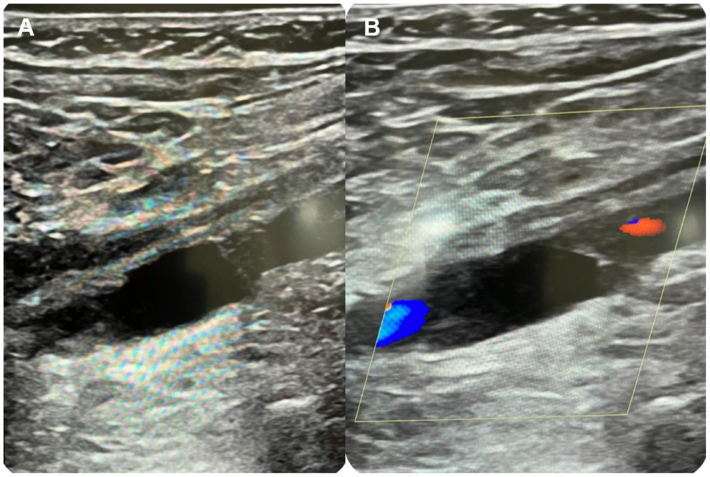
Duplex ultrasound (DUS) of the right popliteal artery confirming cystic adventitial disease (CAD). (A) Longitudinal B-mode image demonstrating an anechoic, cystic structure compressing the arterial lumen. (B) Color Doppler image showing no-flow within the compressed lumen due to extrinsic compression by the cyst.
